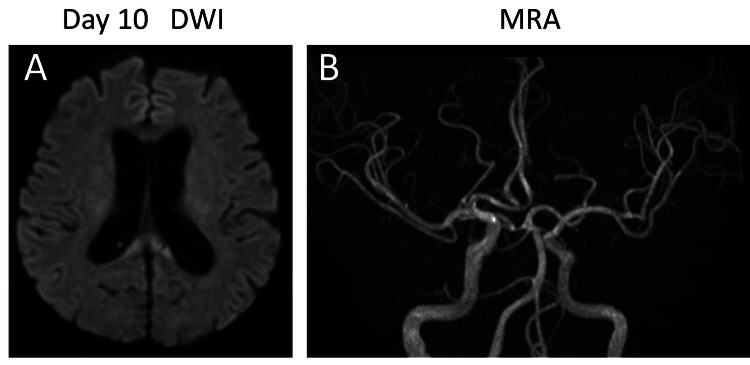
Postoperative images Angiographical vasospasm and symptomatic delayed cerebral ischemia were not observed in DWI (A) and (MRA) DWI: diffusion-weighted imaging; MRA: magnetic resonance angiography
